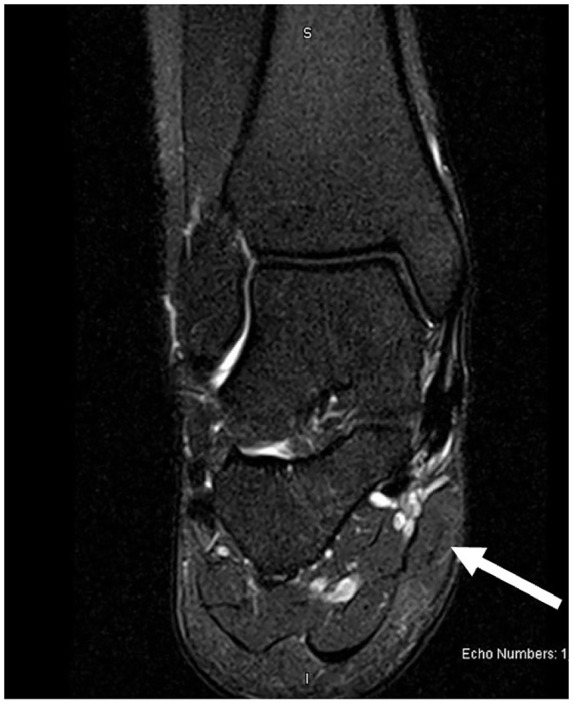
Magnetic resonance imaging: coronal view.
